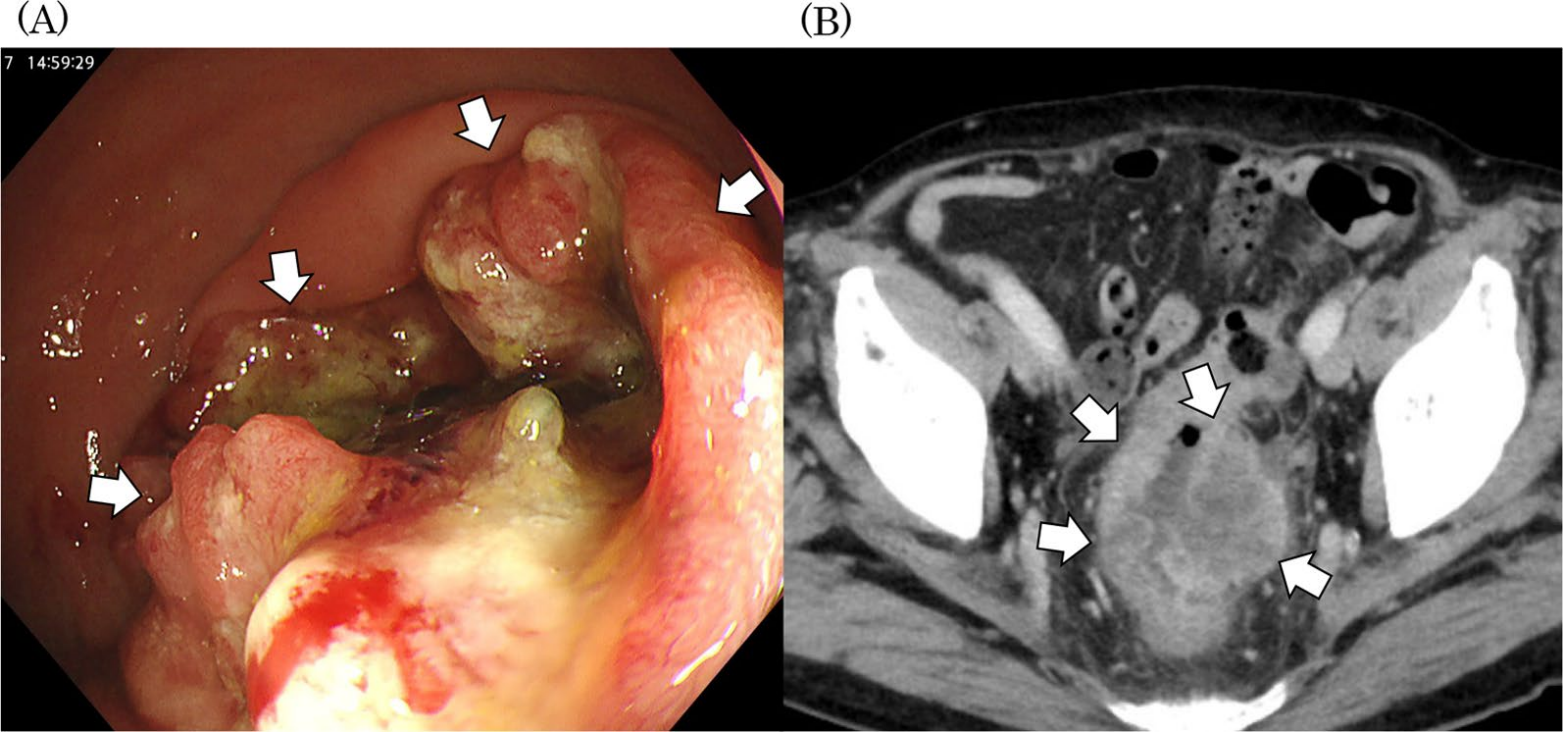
Baseline imaging for Case 1. A Endoscopic imaging showing a rectal tumor 7 cm from the anal verge. B Computerized tomography (CT) scan staging a locally advanced rectal cancer (cT4aN1bM0). Arrows identify the tumor
